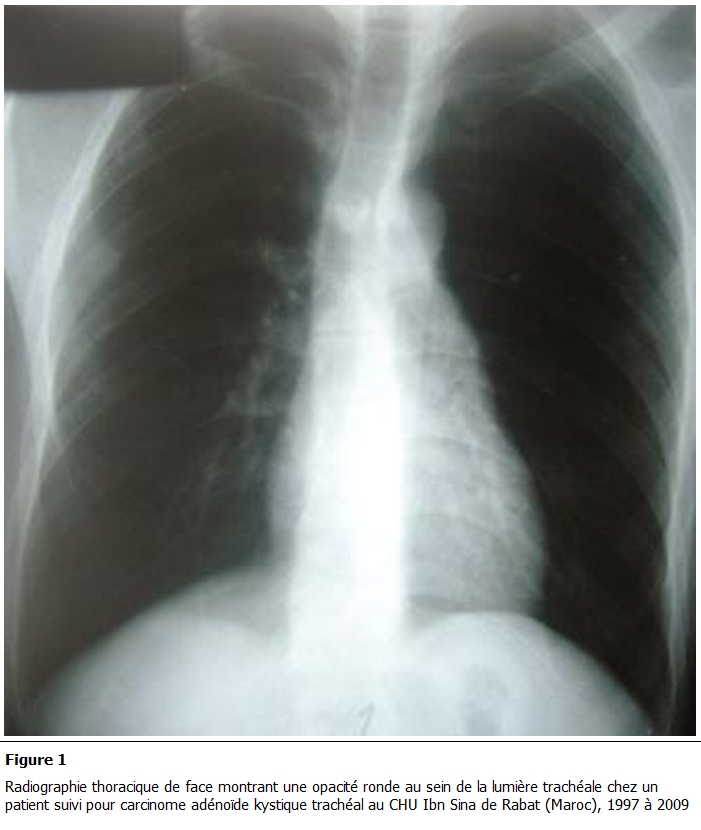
Radiographie thoracique de face montrant une opacité ronde au sein de la lumière trachéale chez un patient suivi pour carcinome adénoïde kystique trachéal au CHU Ibn Sina de Rabat (Maroc), 1997 é 2009
